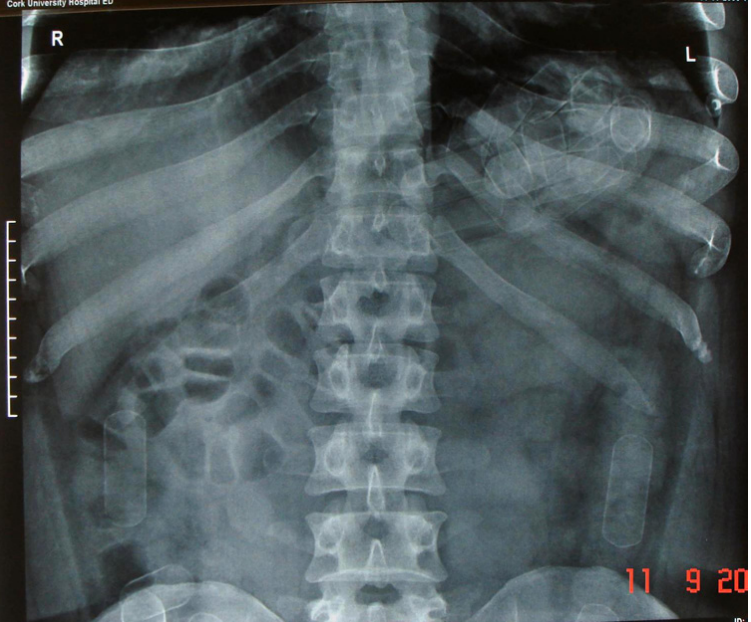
Plain radiograph of the abdomen demonstrated multiple homogenous radiopacities within the lumen of the bowel demonstrating the typical appearances of Type IV packages.
